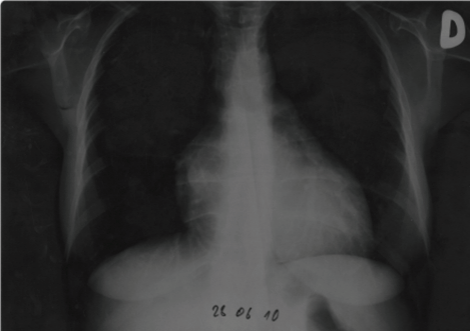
Chest radiography revealed heart enlargement
